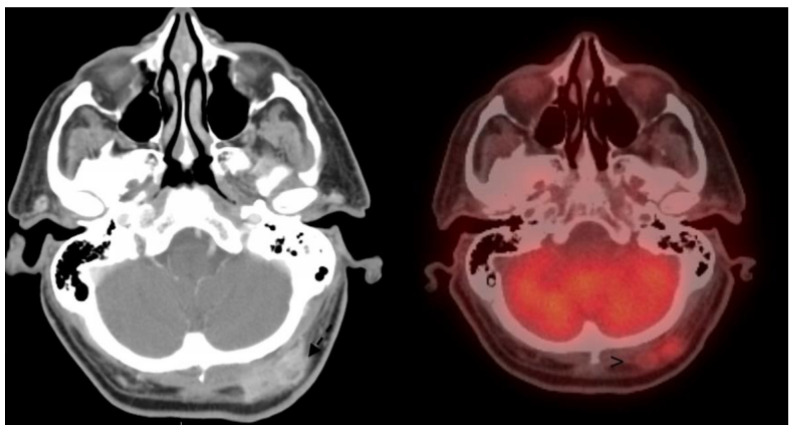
Axial contrasted computed tomography (CT) of the head on (left) with involved occipital node from a primary scalp squamous cell carcinoma, with positive fluorine-18-fluorodeoxyglucose positron emission tomography/computed tomography (FDG-PET/CT) as seen on (right).
